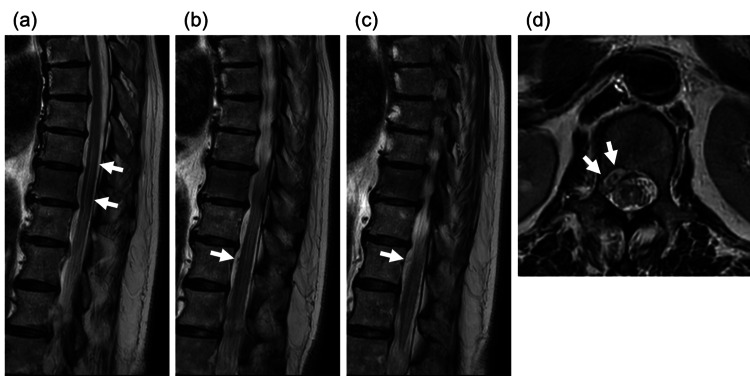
Sagittal T2-weighted images of the thoracolumbar spine (a-c) revealed long-segment intramedullary T2 hyperintensity extending from T12 to conus (a, arrows), indicating cord edema from venous congestion. Note the serpiginous perimedullary flow voids along the dorsal surface of the cord (b-c, arrows), which are suggestive of dilated perimedullary veins and should raise suspicion for a spinal arteriovenous fistula. Axial T2-weighted image (d) demonstrated an enlarged epidural venous plexus ventral to the spinal cord from T12 to L3 (arrows), a key finding in spinal epidural arteriovenous fistula that reflects the abnormal venous drainage pattern.
